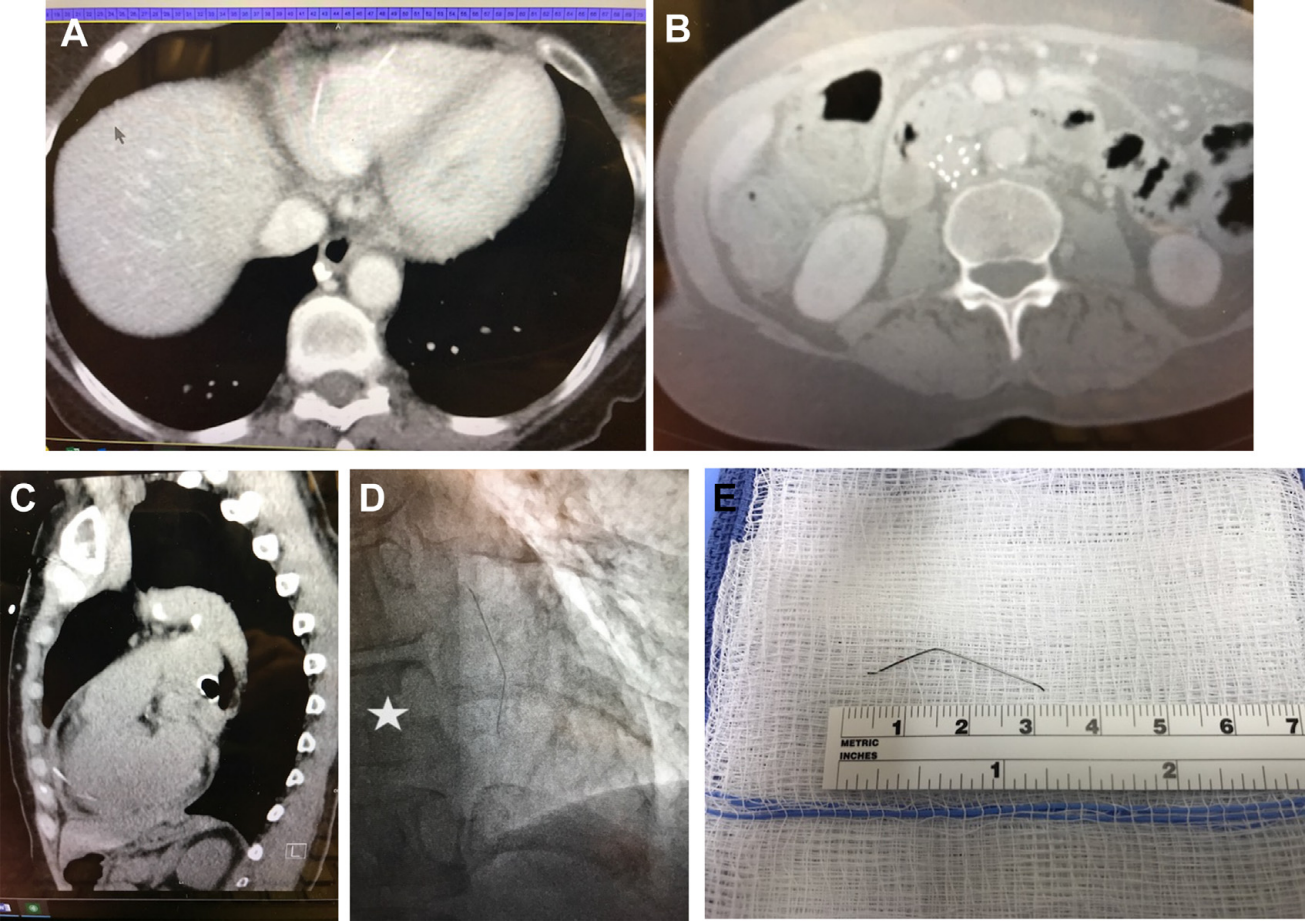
Computed tomography angiography of the chest and abdomen demonstrates (A) a metallic fragment (arrow) embedded in the anterior right ventricular free wall without a pericardial effusion and (B) an inferior vena cava filter that had only 11 struts when there should be 12. (C) Subsequent gated computed tomography angiography of the chest demonstrates further migration of the fractured strut, which is now penetrating the right ventricular free wall and extending into the pericardial sac with a new small effusion. (D) At the time of operation, a lateral chest radiograph demonstrated further migration of the strut into the posterior pericardium parallel to the spine (star). (E) After a limited left anterior lateral thoracotomy and pericardiotomy, the fractured strut was removed uneventfully.
